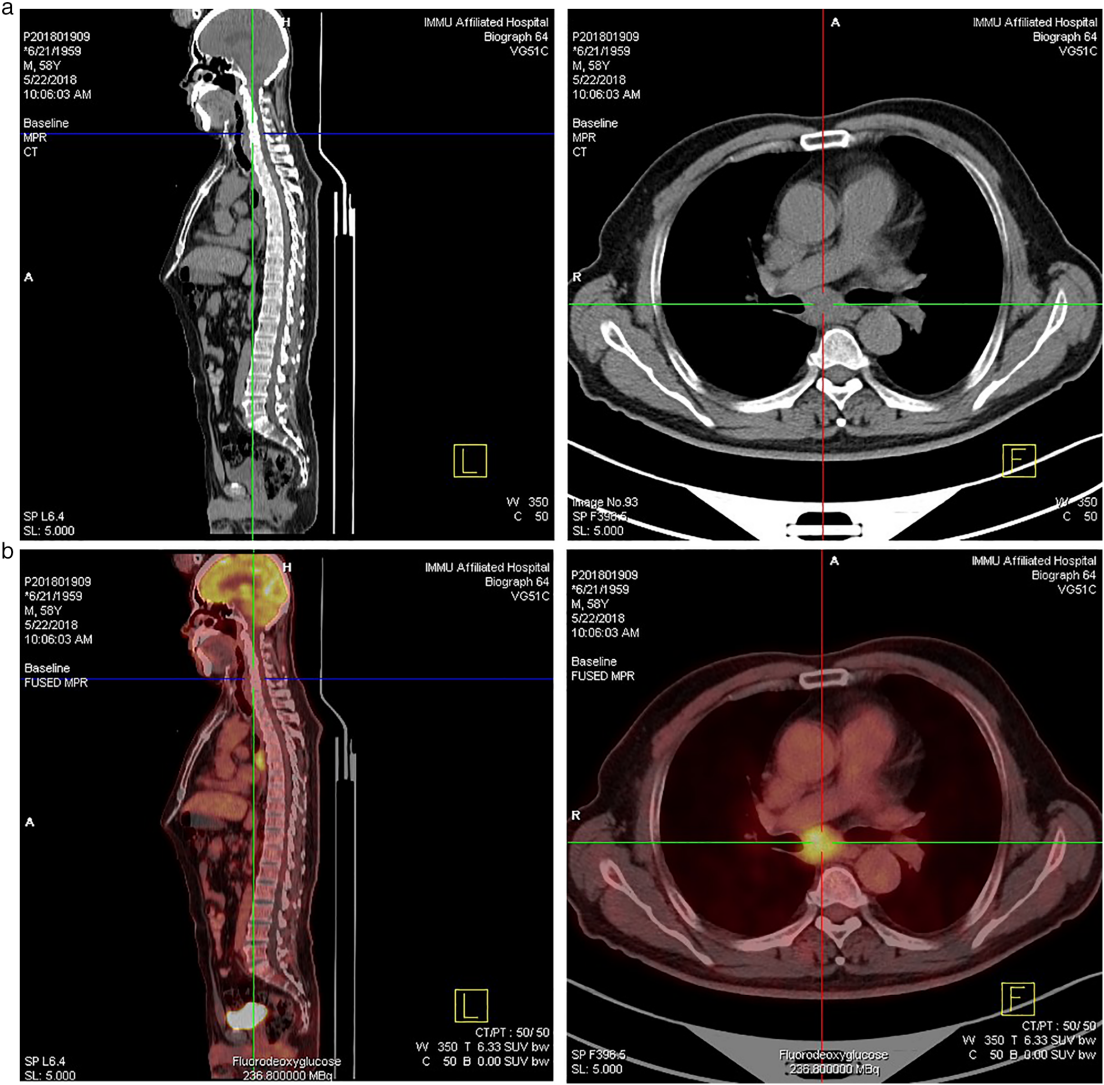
(a) PET‐CT showed multiple enlarged lymph node shadows associated with increased metabolism in the anterior tracheal vena cava, right pulmonary hilum and subcarina. (b) [(18)F] fluoro‐2‐deoxy‐D‐glucose positron emission tomography/computed tomography imaging revealed an area of abnormal FDG accumulation in the mediastinum.
